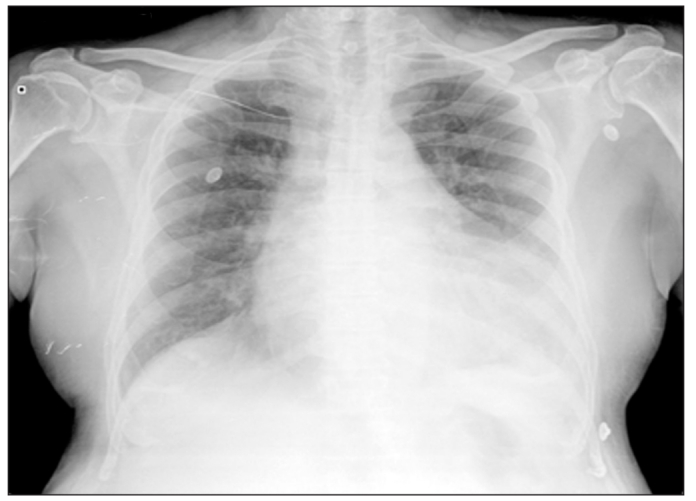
Preoperative chest radiograph
